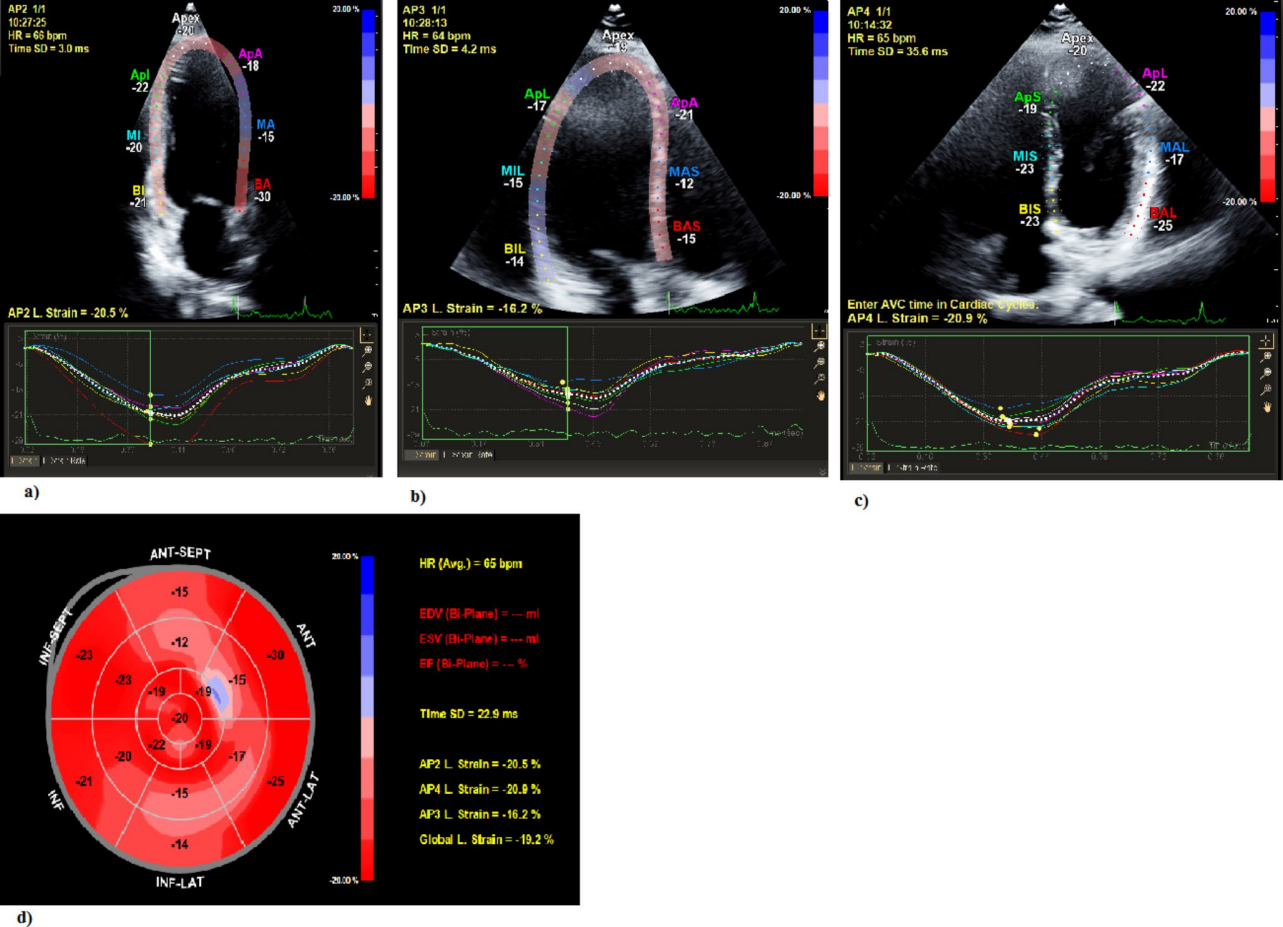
Measurement of global longitudinal strain (GLS) by speckle tracking analysis in an obesity patient (45 year old female, BMI 38.4 kg/m^2^). a) Apical 4-chamber view with measurement of longitudinal strain. b) Apical 2-chamber view with measurement of longitudinal strain. c) Apical 3-chamber view with measurement of longitudinal strain. d) Bull’s eye graph showing longitudinal strain for all myocardial segments, of which a weighted mean was used to derive GLS.
